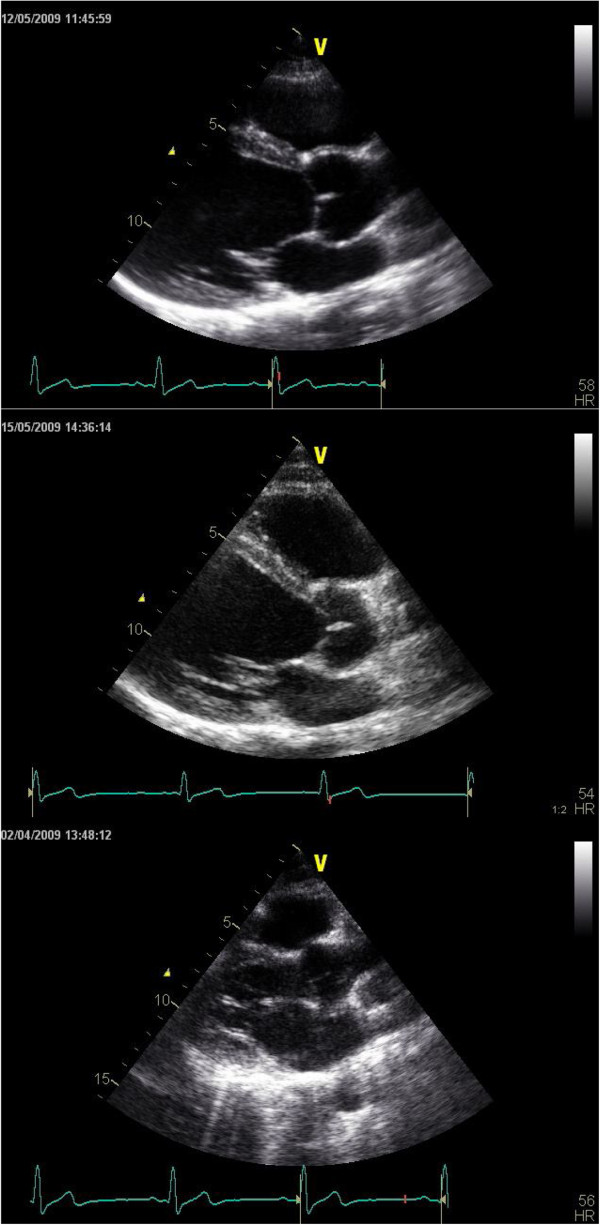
Grading the 2D parasternal long axis (PLAX) view. The grading includes anatomical presentation, use of screen and use of technical settings. This figure shows a PLAX image from an expert (top) an intermediate (middle) and a novice (bottom). The blinded scores for the anatomical presentation was expert 5 (very good), intermediate 3(adequate) and novice 1(very poor), for use of screen 5 (very good), 4(good) and 2(poor), and for use of technical settings 4(good), 4(good) and 3(adequate). All scores were finally added up to a total checklist score.
